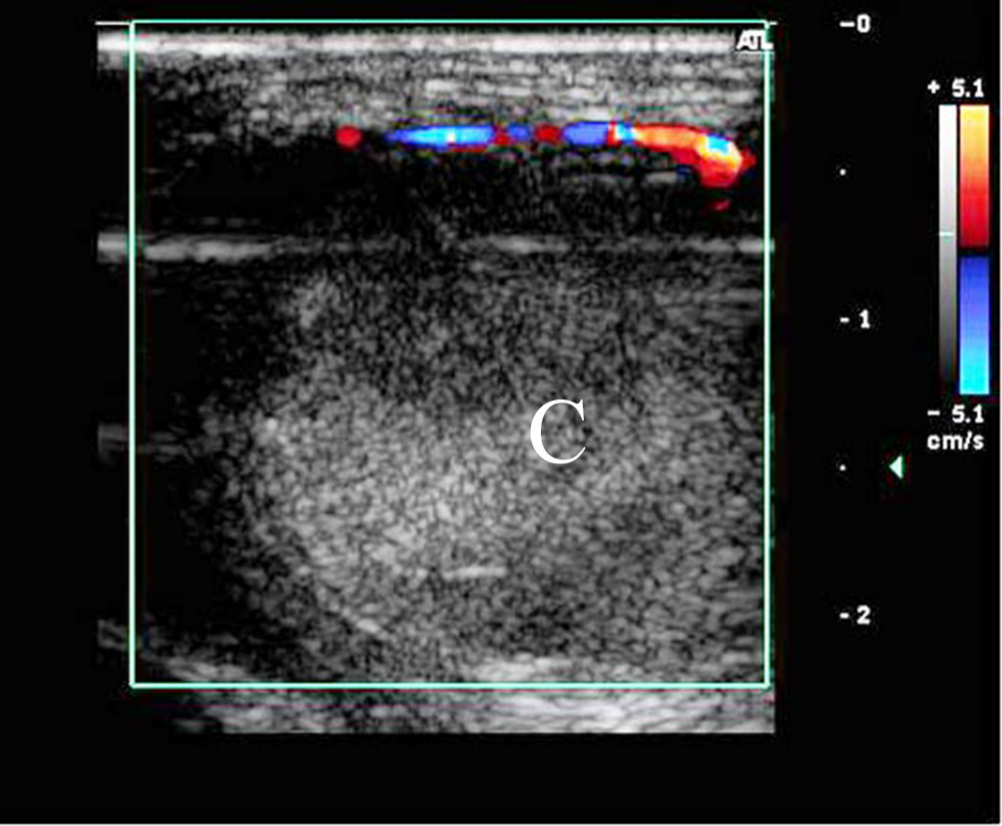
Tranverse sonographic view of the pseudoaneurysm after injecting thrombin (5 units). It shows complete and abrupt cessation of flow in the colour Doppler image within the lesion owing to clot (C) formation.
